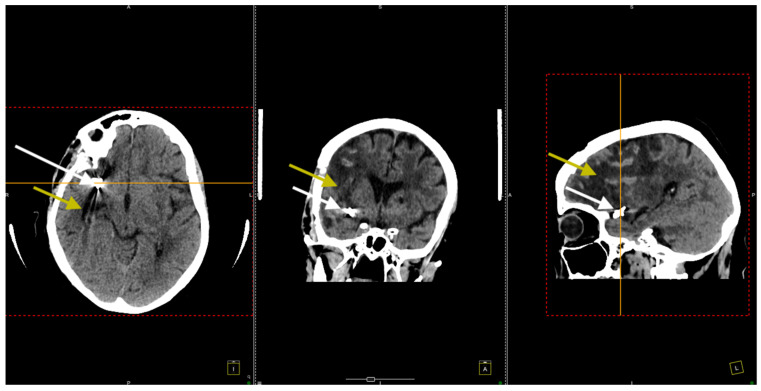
The CT scan conducted 3 months after the surgical procedure. All three sections, transversal (left image), coronal (middle image), and sagittal (right image), confirmed the right frontotemporal hypodensity consistent with ischemic stroke (yellow arrows) and noted artifacts from the previously placed aneurysm clips (white arrows).
